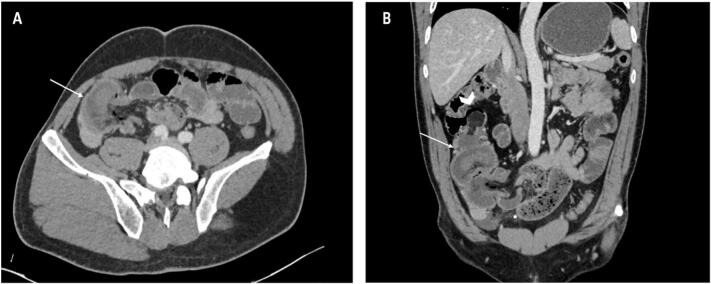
Axial (A) and coronal (B) view of abdominal computed tomography scans demonstrate an ileocolic intussusception (arrow). The leading structure was a fatty dense structure within the bowel lumen that was separate from the mesentery (arrow head). There is a pneumoperitoneum due to small bowel wall necrosis.
